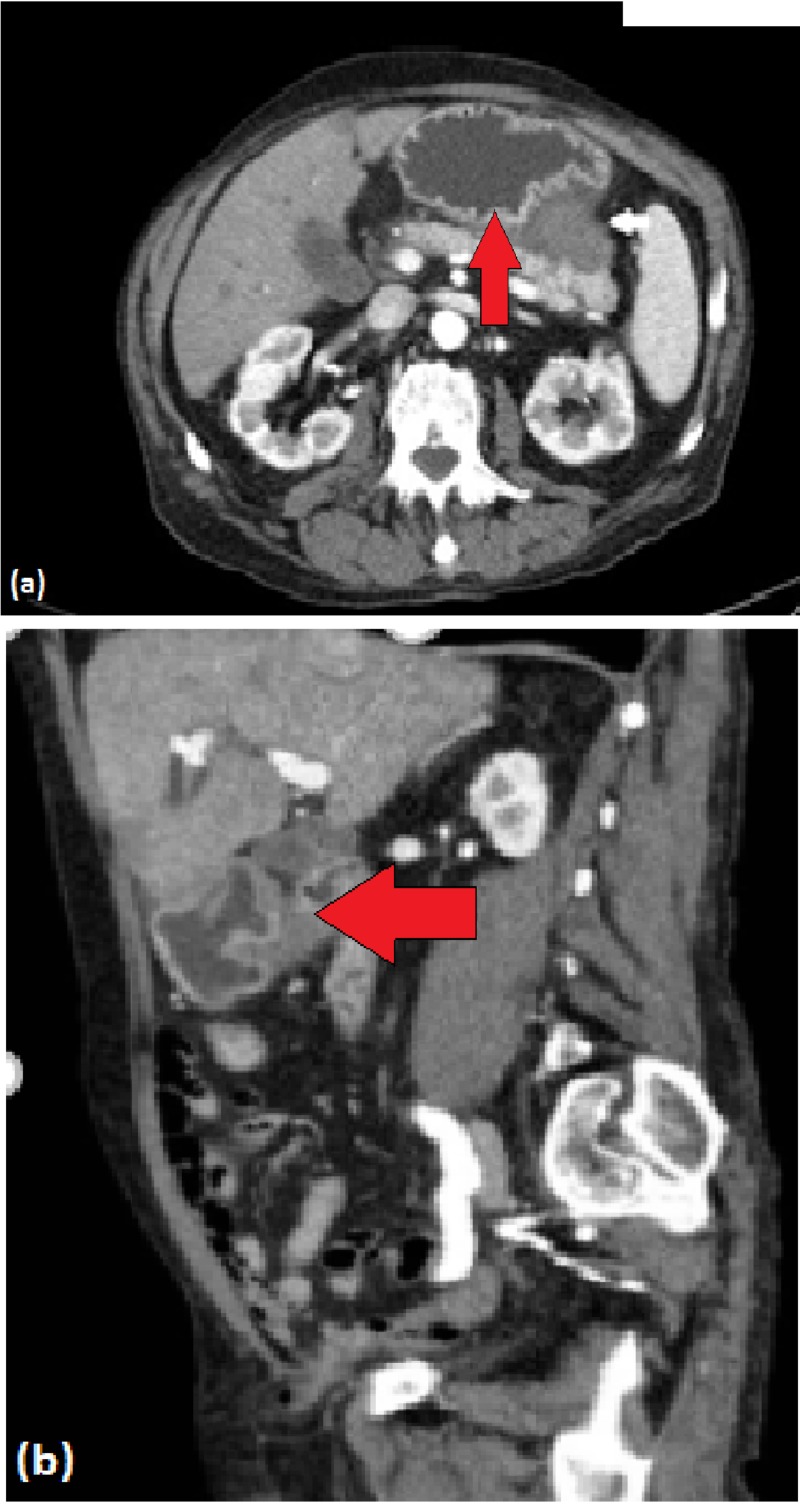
Computed tomography of complex pancreatic mass measuring 5.5 x 4.9 x 4.3 cm, which asserts mass effect along the proximal greater curvature of the stomach towards the fundus (red arrows). (a) Axial view. (b) Coronal view.
